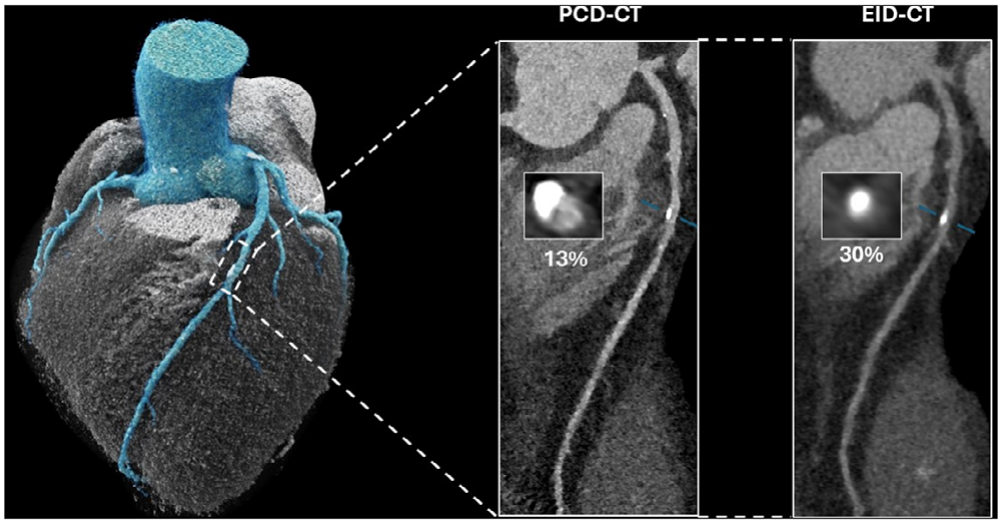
Stenosis of the middle left anterior descending artery in a 69‐year‐old male. Overestimation of the diameter stenosis on the energy‐integrating detector (EID)‐CT compared to the photon‐counting detector (PCD)‐CT using the ultrahigh‐resolution detector mode and reconstructed to a slice thickness of 0.2 mm, is most likely due to extensive blooming artifacts which obscure the true lumen boundary.
